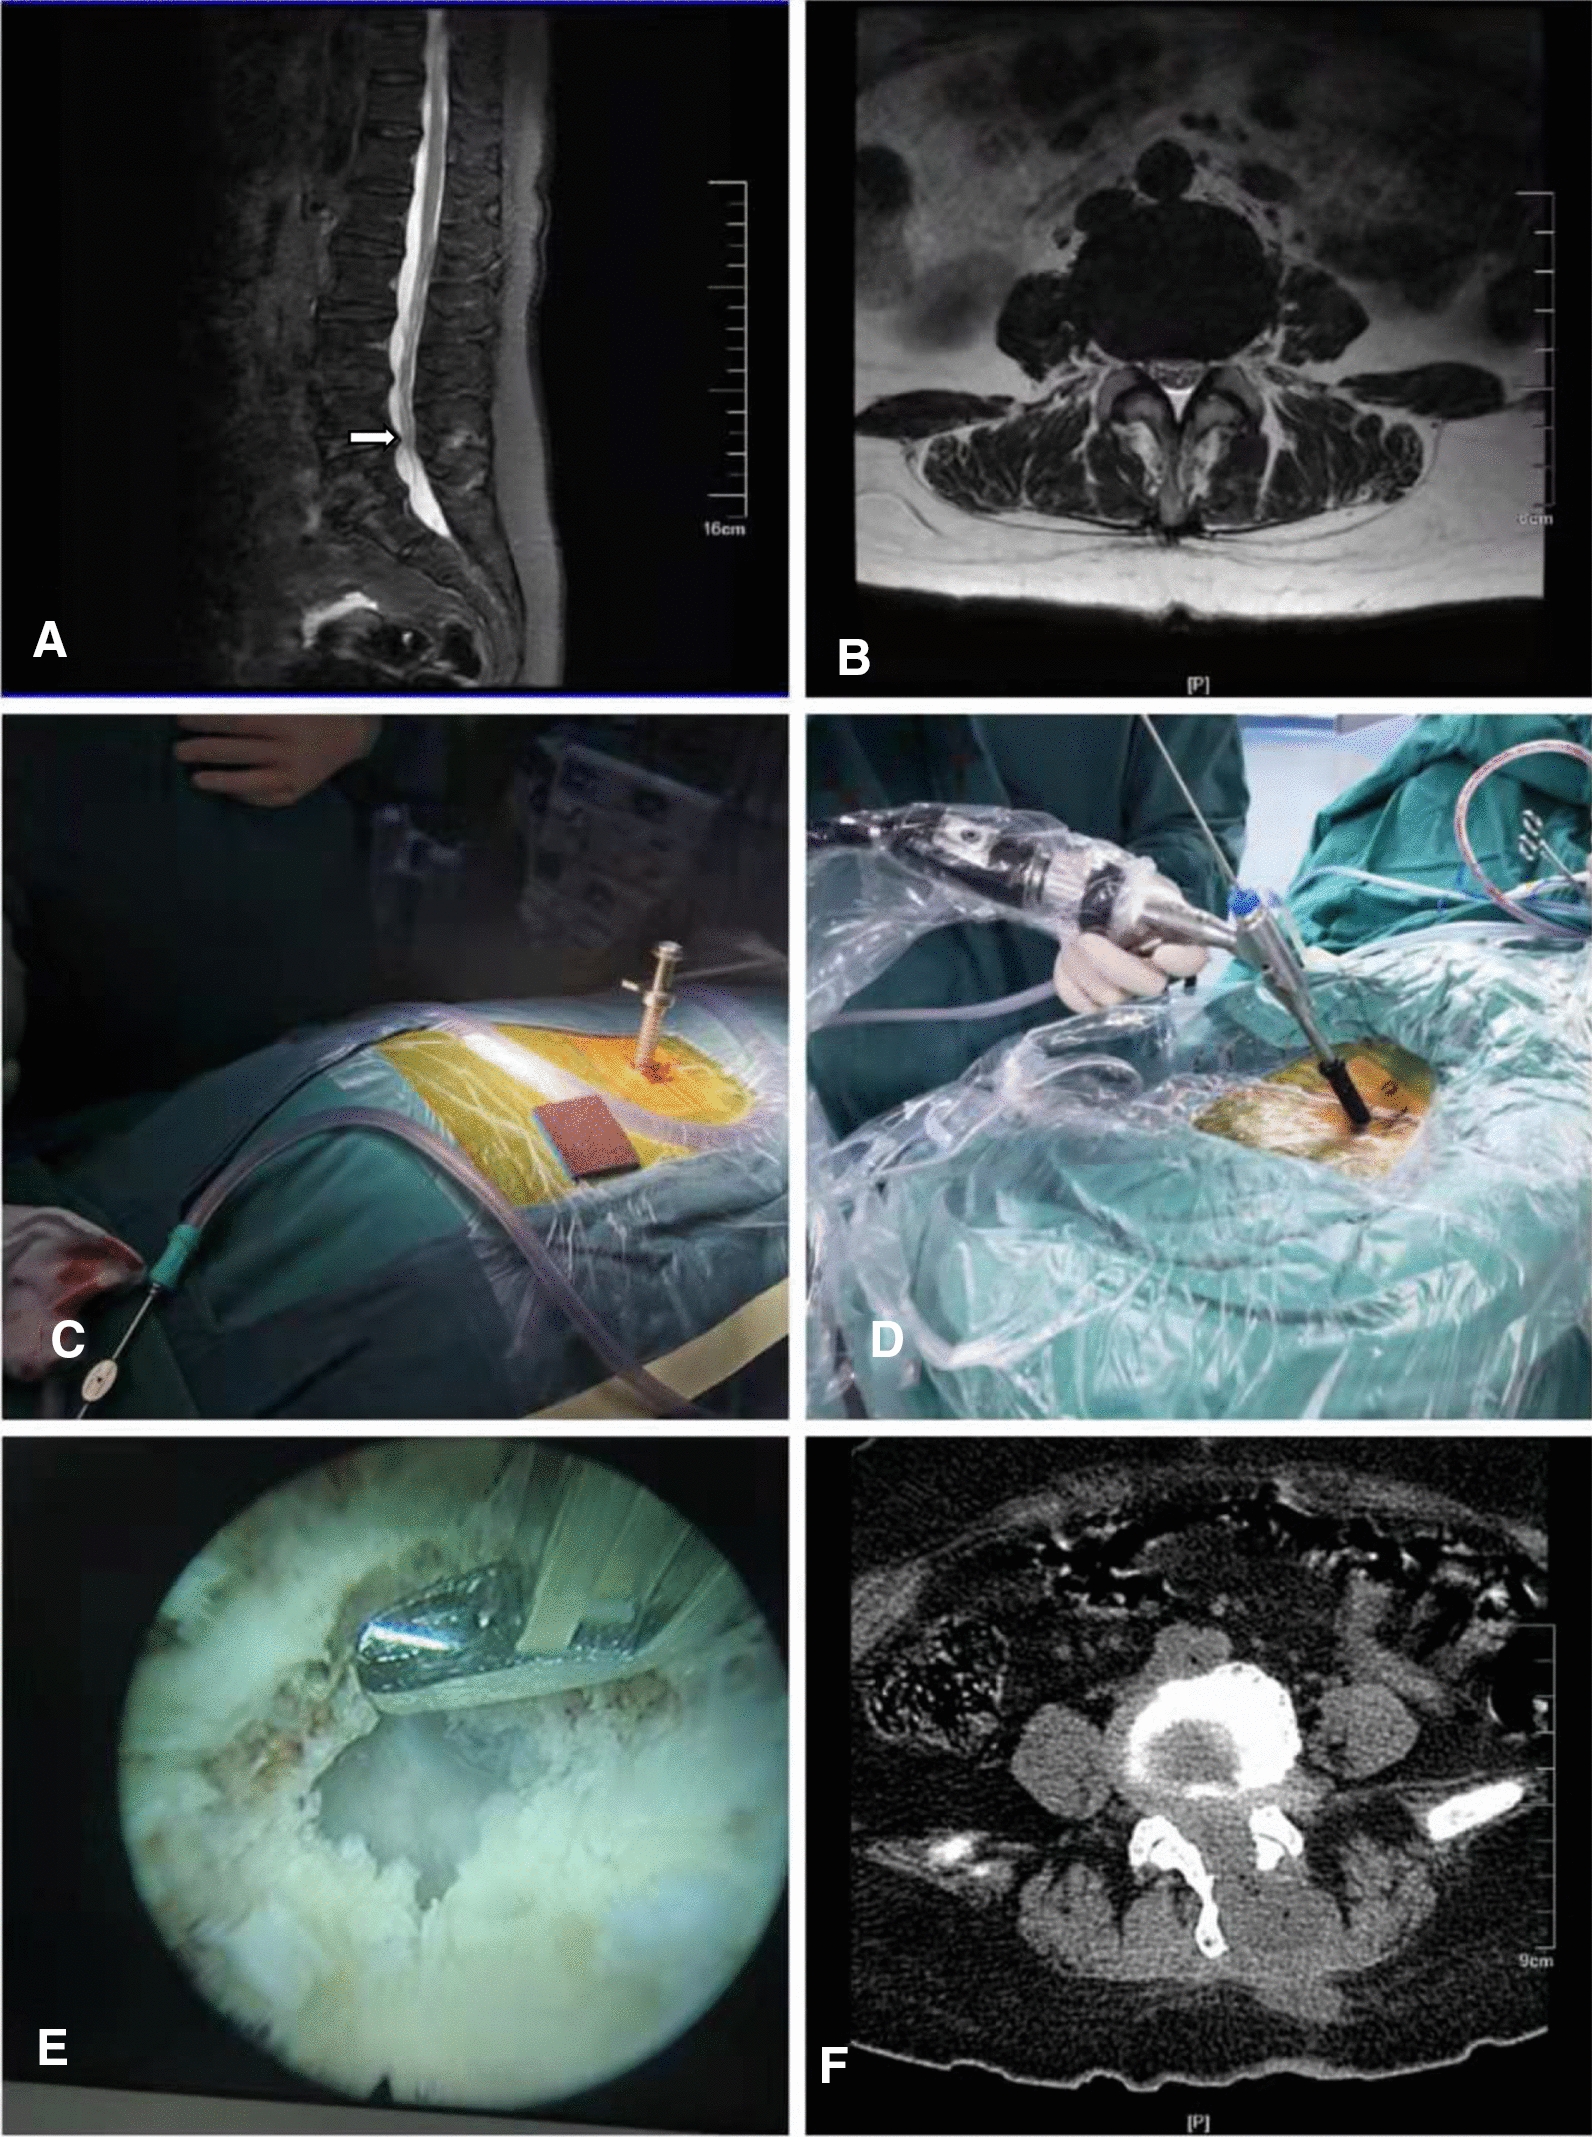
The imaging data of a 76-year-old woman with LSS treated by Delta large channel endoscopy. A, B Preoperative MRI showed L4/5 spinal canal stenosis. C, D Patient's position and established operating channel. E Exposure of the nerve roots and dural sac of the L4/5 segment. F Postoperative cross-sectional CT showed adequate decompression of the L4/5 spinal canal. White arrow indicates level of decompression
